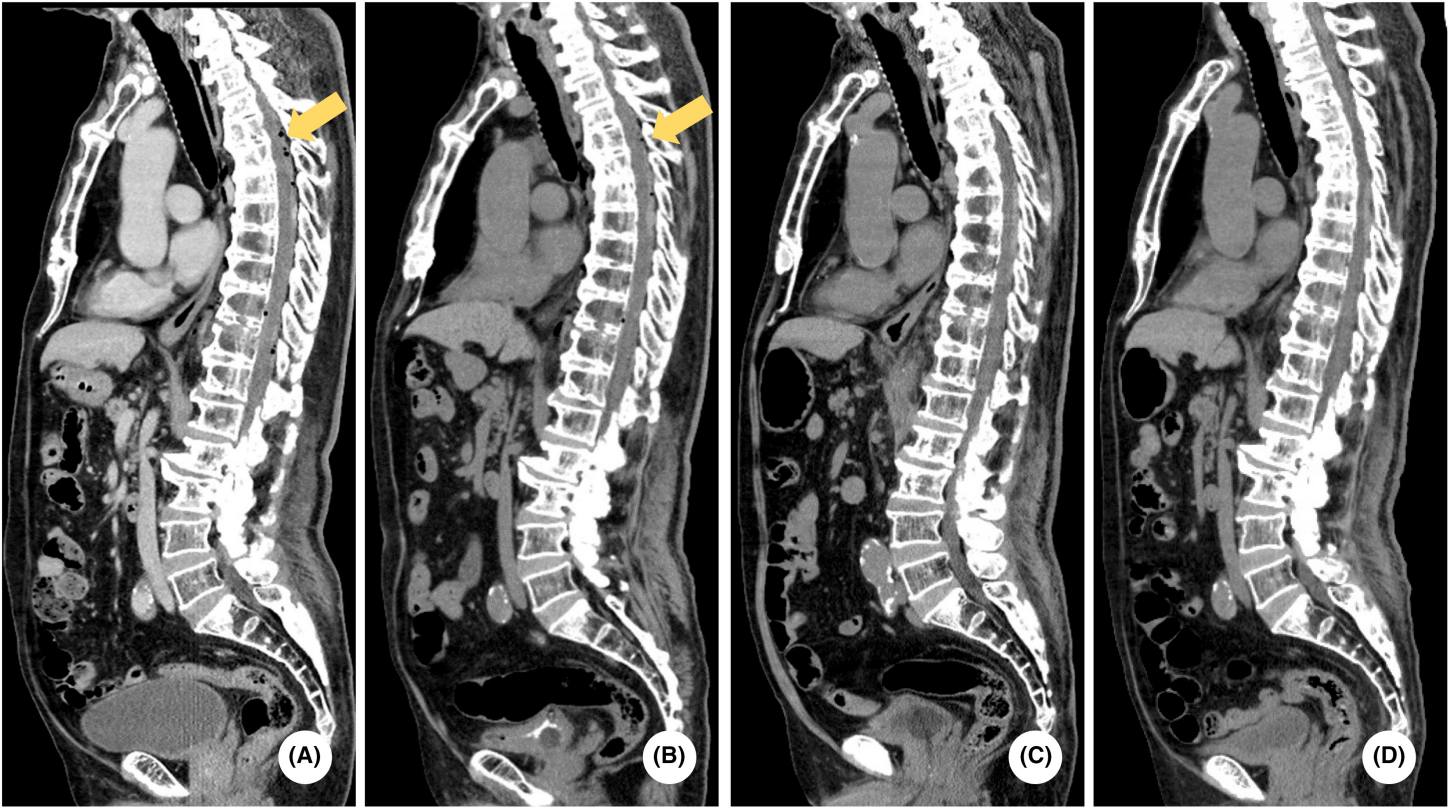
Computed tomography (CT) of the patient’s spine. Pneumorrhachis (yellow arrows) is shown in A and B of the spinal CT image. A：Day 1, B：Day 2, C：Day 4, D：Day 9.
